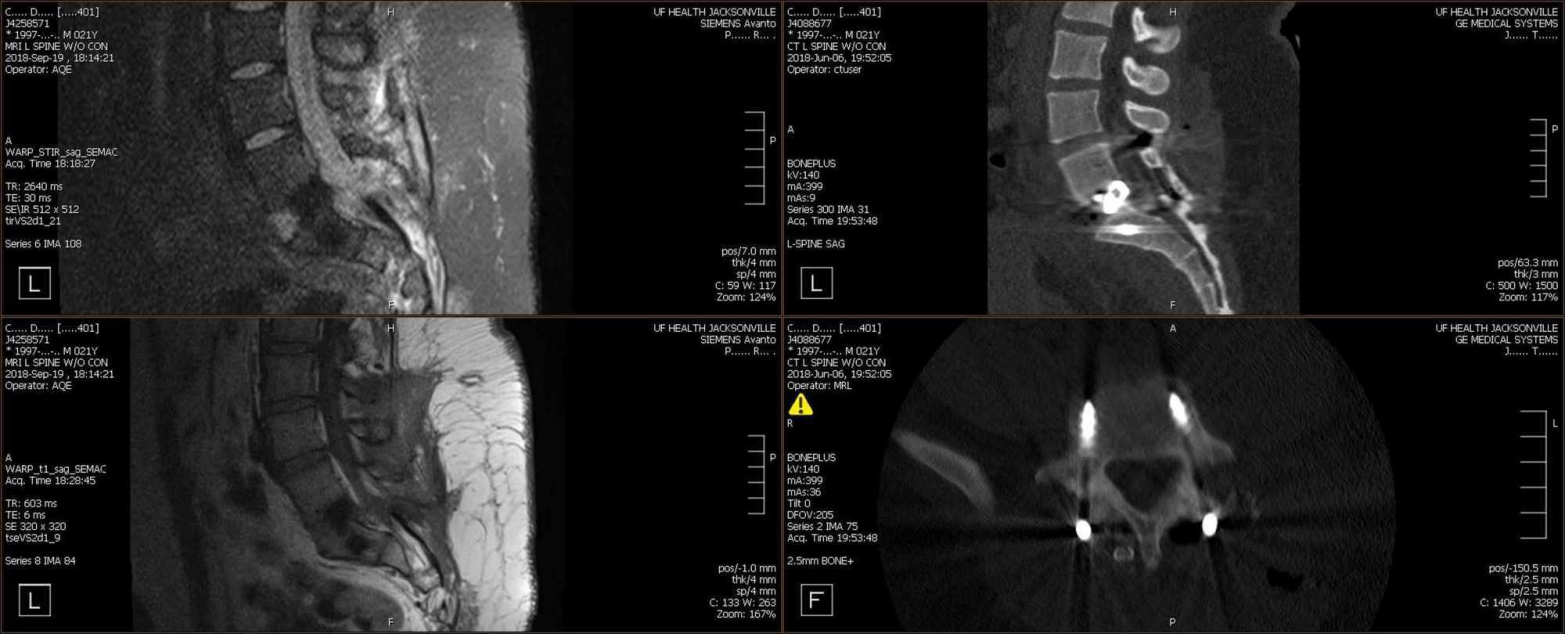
Postoperative follow-up magnetic resonance imaging (MRI - left upper and lower) and computed tomography images (CT - right upper and lower quadrants) MRI follow-up at three months, with the canal widely decompressed and an evident defect at L5-S1 without any evidence of a pseudomeningocele. CT scans revealed anterior and posterior implants in place.
